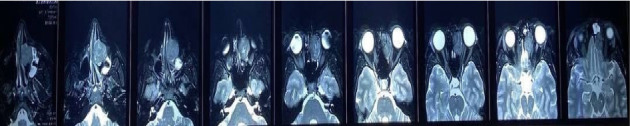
The T2-weighted MRI of presented case.
